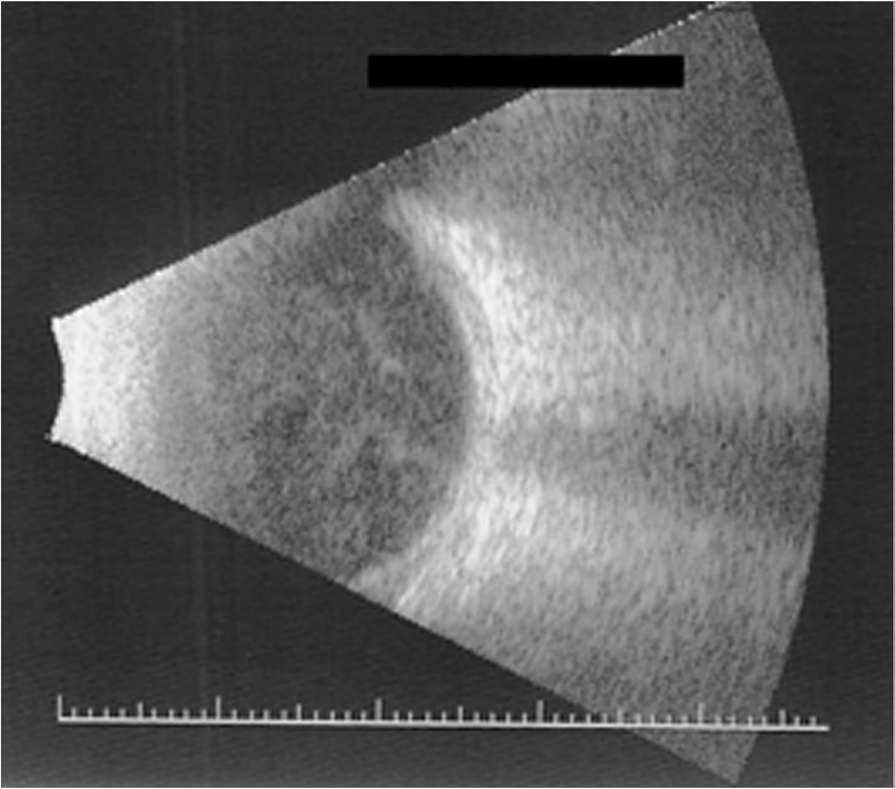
Infiltration of the vitreous on the second day after injury (ocular ultrasound)
